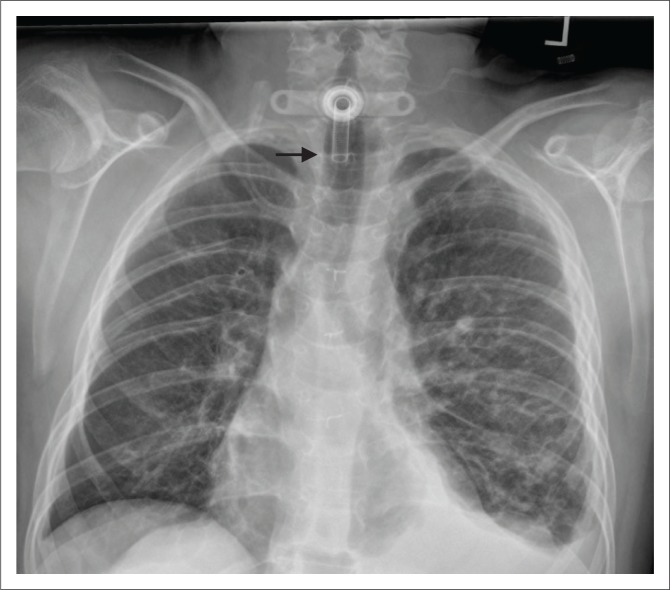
Chest radiograph showing an accurately positioned tracheostomy tube (arrow).
